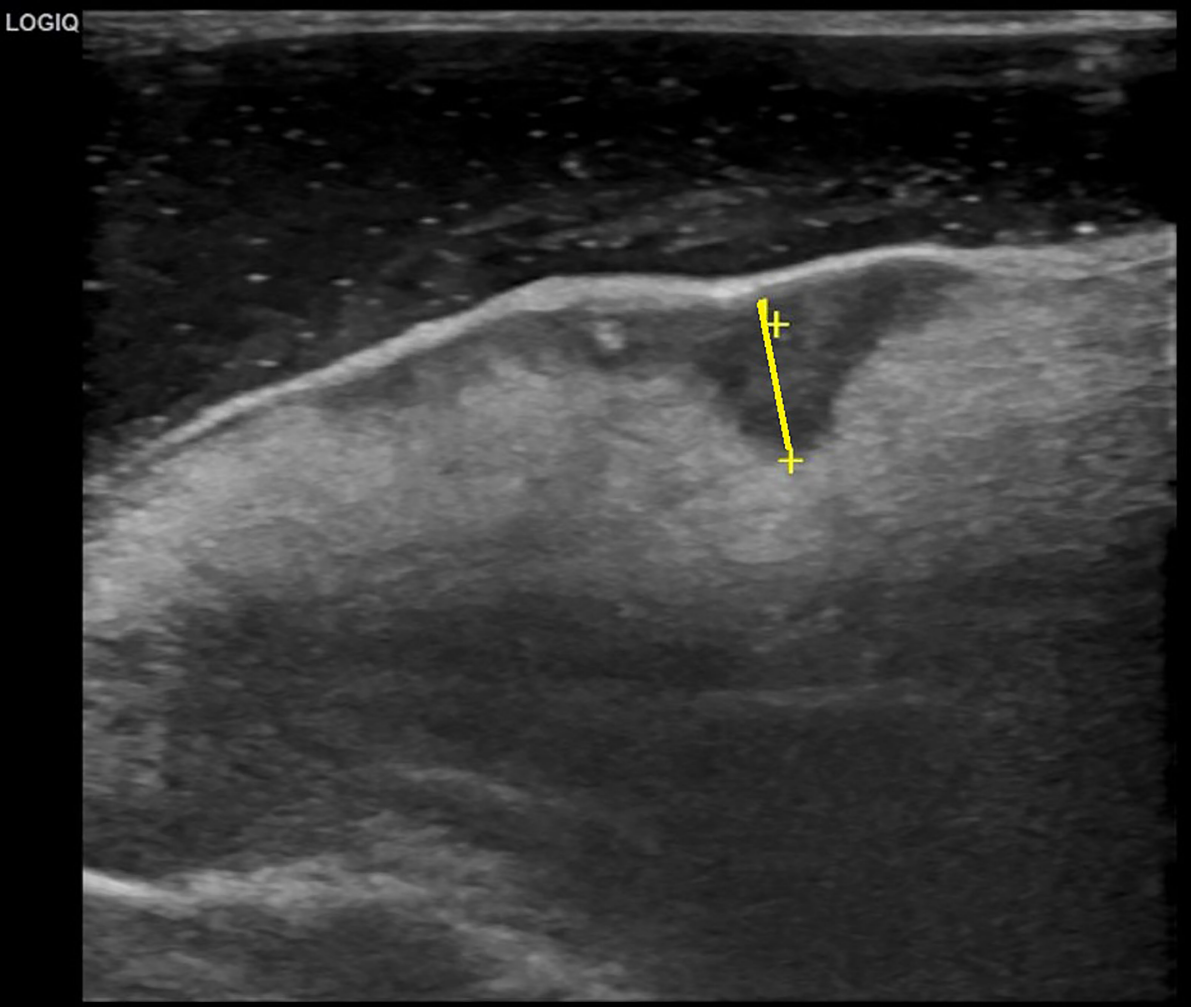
Measurement of sonographic depth of invasion (yellow line).
